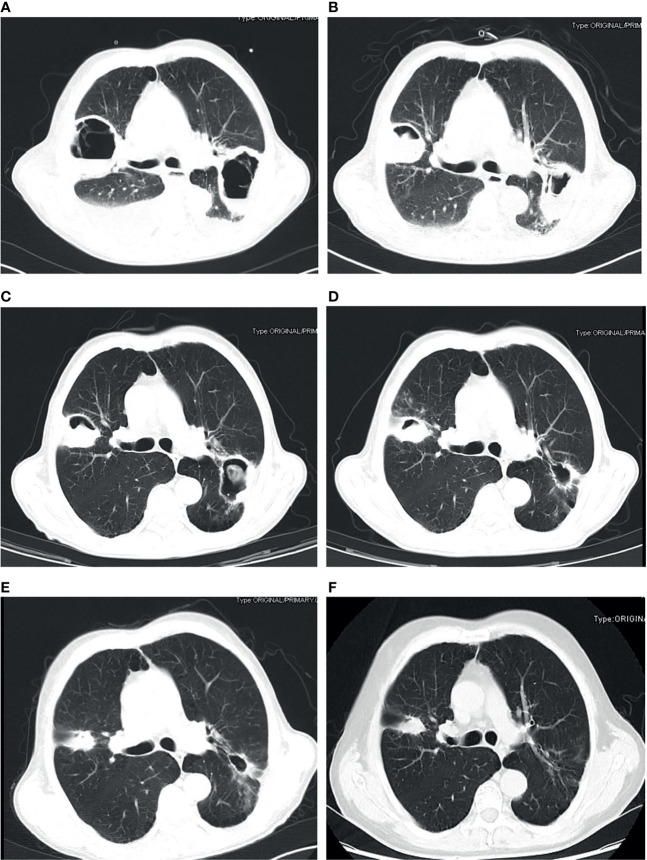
Pulmonary fungal infection (*Mucor* with *Aspergillus*) in a 69-year-old man with uncontrolled diabetes, chronic obstructive pulmonary disease and liver cirrhosis. CT imaging showed thick-walled cavity shadow with gas-fluid level in the right upper lobe and left lower lobe of the lung (A). The lesion was significantly reduced after half a month of treatment with posaconazole (B). The lesion continued to shrink after 40 days (C), three months (D), five months (E) and 1 year (F) of treatment.
